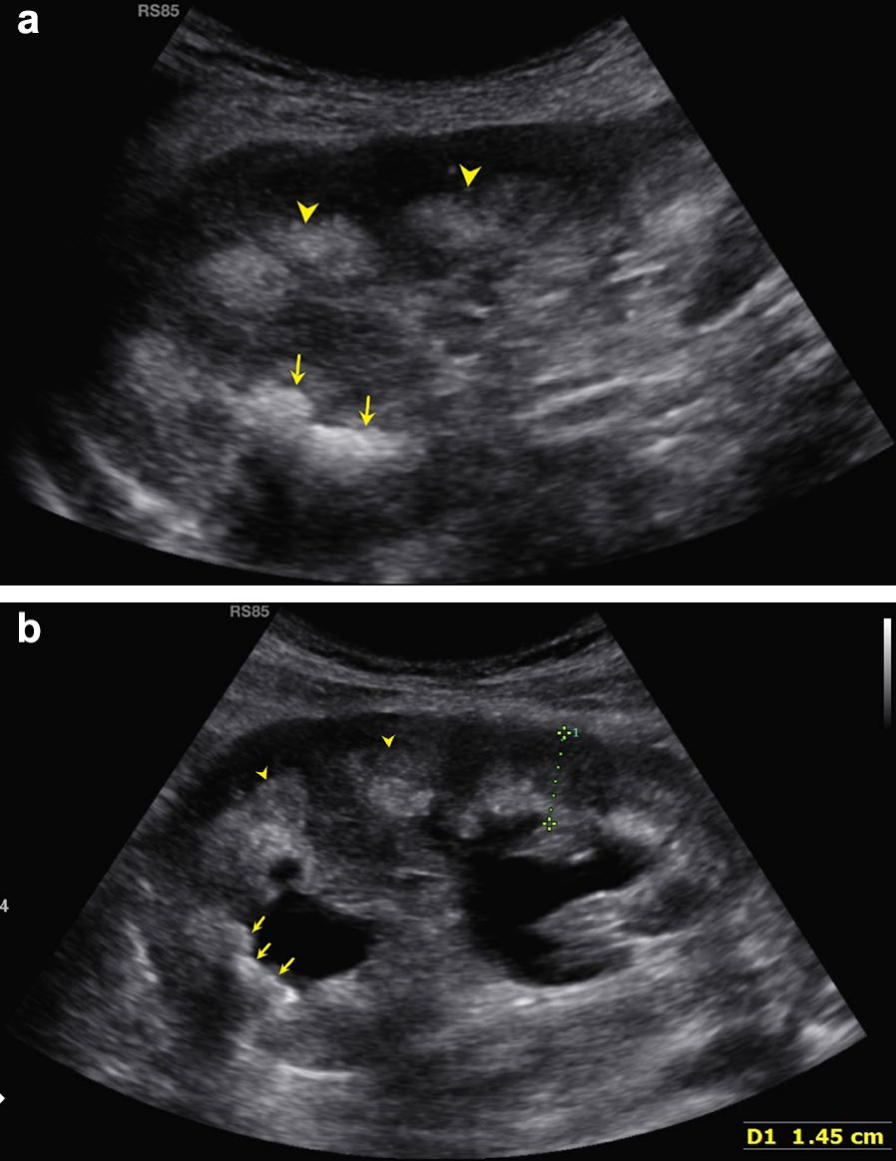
Philips EPIQ, convex transductor, B-mode, frequency 2,5–5 MHz, right lower abdomen quadrant. a The caudal kidney shows a hypoechoic cortical with hyperechoic medulla (arrowheads). The superior calyceal group presents even large kidney stones with the typical rear shadow cone behind (arrows). b The cranial kidney shows the same echographics characteristics of the caudal kidney: a hypoechoic cortical with hyperechoic medulla (arrowheads), and kidney stones with rear shadow cone (arrows). Moreover, we can appreciate the dilatation of renal pelvis probably due to bladder replenish or urethral stones. Renal parenchymal thickness (among calipers) is normal suggesting a preserved renal parenchyma. Both kidneys present the same sonography pattern, consistent with medullary sponge kidney (MSK)
